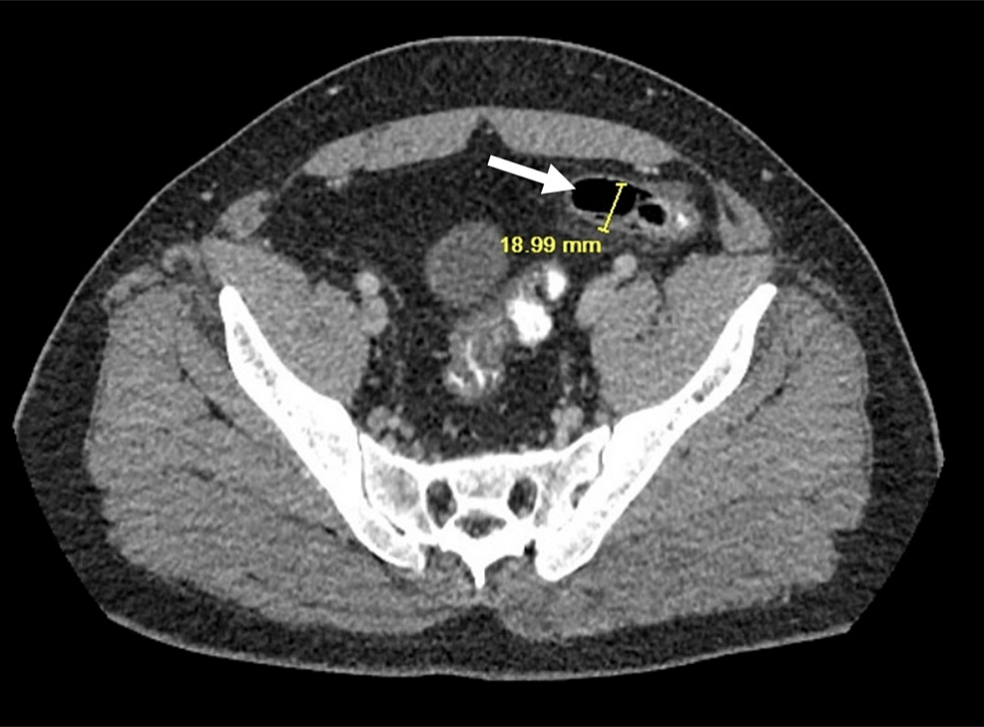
CT scan showing left-sided appendicitis (white arrow).
